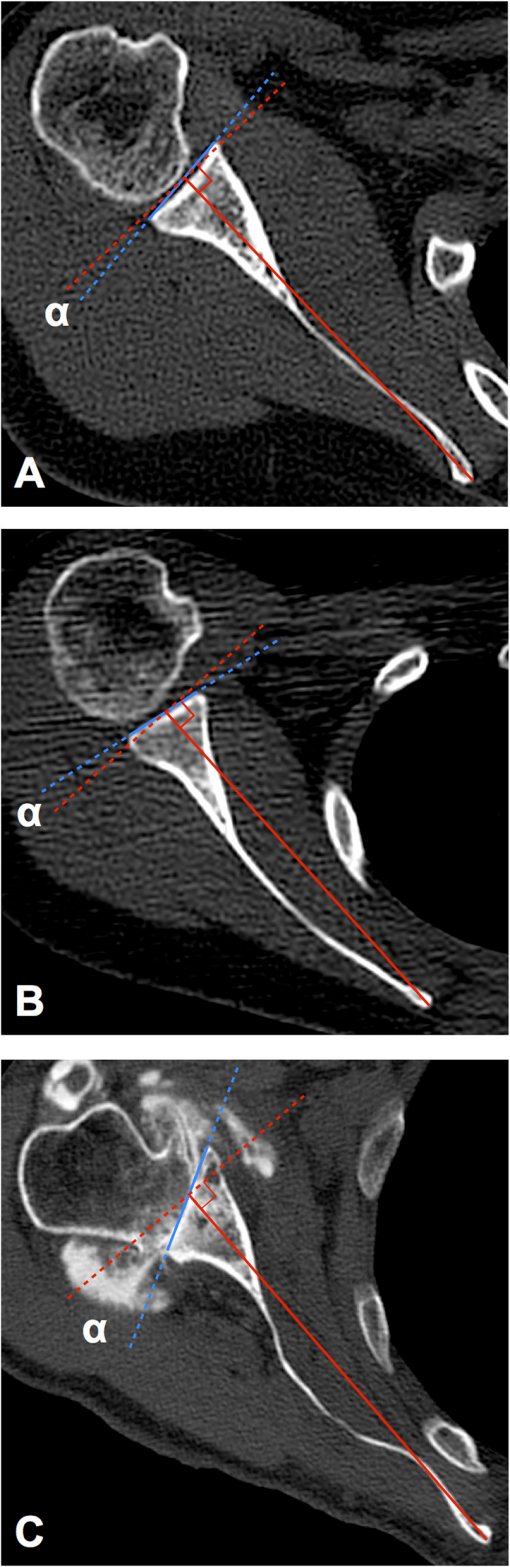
The conventional method for glenoid version measurement. The figures describe the conventional measuring method for glenoid version described by Friedman et al. The glenoid line is defined as the line connecting the anterior and the posterior rim of the glenoid (*blue line*). The scapular axis is defined as the line connecting the root of the scapular spine and the center of the glenoid line (*red solid line*). Glenoid version (*α*) is calculated as the angle between the glenoid line and the line perpendicular to the scapular axis (*red dashed line*). (A) The conventional method for measuring a normal shoulder. The scapular body shape appears flat. The glenoid shows 6.2° retroversion. (B) The conventional method for another normal shoulder. The scapular body shape is round. The glenoid shows 8.9° anteversion. The conventional method is sensitive to the scapular body shape that varies widely between patients. (C) The conventional method for measuring an arthritic shoulder. The scapular body is waving. CT arthrograms are used to measure glenoid version, and the glenoid has 28.3° retroversion. The intermediate line is selected as the glenoid line in the Walch classification B2 glenoid.
